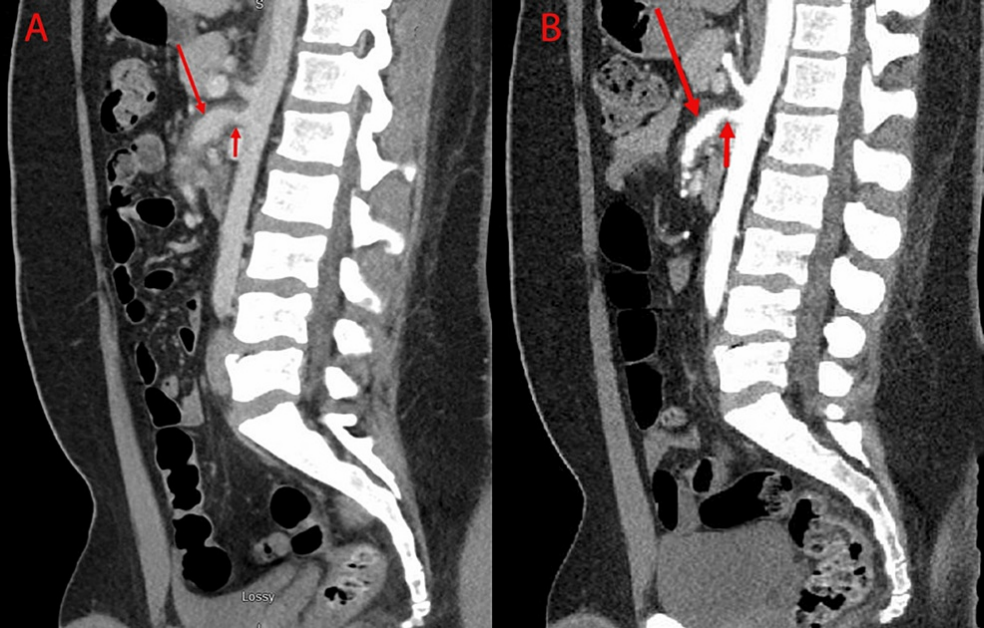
CT scans showing the sagittal sections of the superior mesenteric artery. CT scans showing the sagittal sections of the superior mesenteric artery before (A) and after (B) two months of treatment with steroids. A: CT scan with intravenous contrast showing the narrowing of the superior mesenteric artery (short arrow) followed by 1.5 cm aneurysmal dilation (long arrow). Notice the increased stranding (inflammation) of the vessel wall. B: CT angiography scan two months after treatment with steroids showing slightly improved narrowing of the superior mesenteric artery (short arrow) and aneurysmal dilation (measuring now 1.3 cm, long arrow). Notice the improved stranding (inflammation) of the vessel wall.
